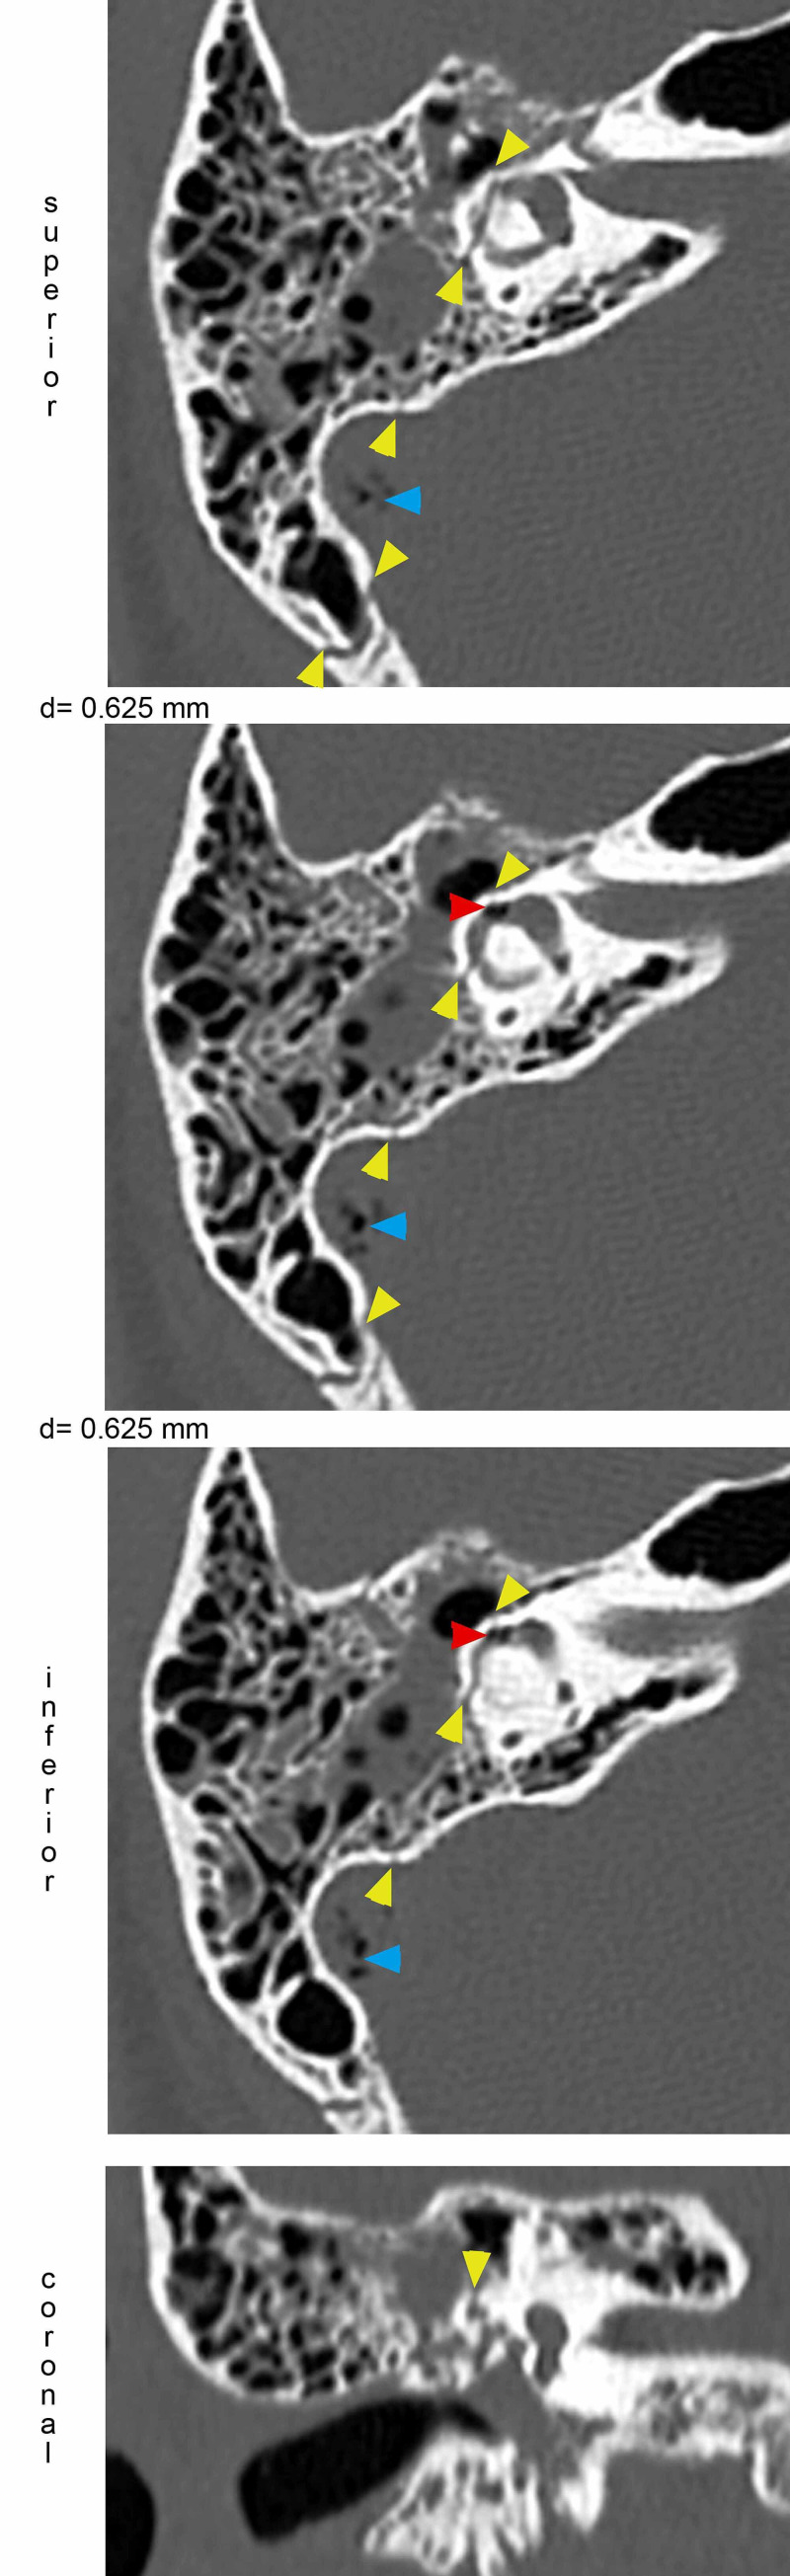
Demonstration of the fracture through the lateral SCC resulting in pneumolabyrinth. Axial CT images demonstrating pneumolabyrinth (red arrowheads) inside the right lateral SCC close to the ampulla. The fracture (yellow arrowheads) has a longitudinal trajectory disrupting the lateral SCC. Involvement of the lateral SCC is also visible in the coronal plane. Air bubbles are also present within the sigmoid sinus. Differences (d) of levels were 0.625 mm each. Section thickness: 0.625 mm. SCC: semicircular canal
